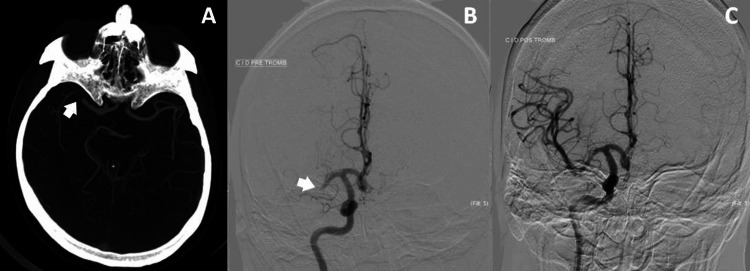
(A) Cranial angio-CT and (B) pre-thrombectomy cerebral angiography documenting an occlusion of the M1 segment of the right middle cerebral artery (arrows); (C) post-thrombectomy cerebral angiography showing reperfusion of the occluded artery
